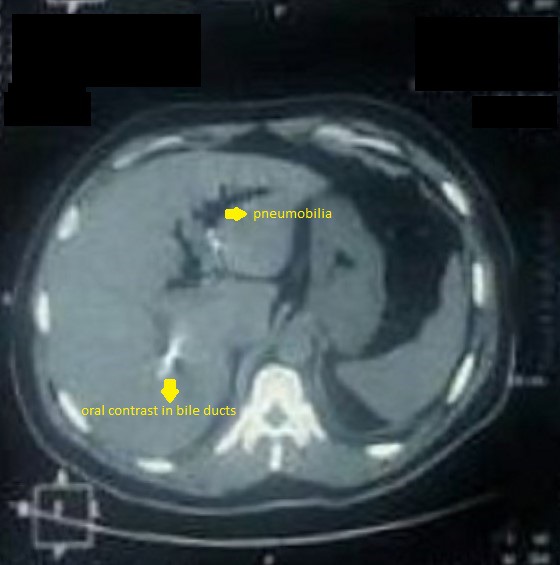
CT scan showing oral contrast in the intrahepatic bile ducts and pneumobilia.
